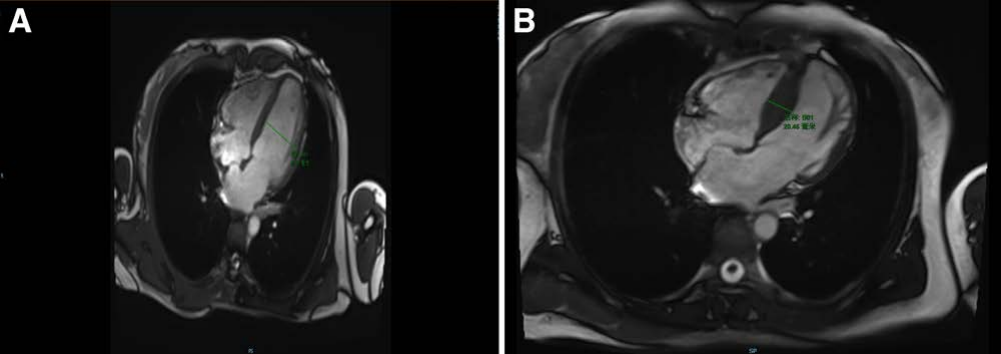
Cardiac MR of the proband and her sister. (A) Cardiac MRI of the proband’s heart: left ventricular systolic function was slightly weakened, with patellar fibrosis of the ventricular septum and lateral wall; (B) cardiac MRI of the second-generation (II3): thickening of interventricular septum (20 mm), the anterior and inferior wall of the left ventricle with left ventricular myocardial fibrosis and focal myocardial edema. MRI = magnetic resonance imaging.
